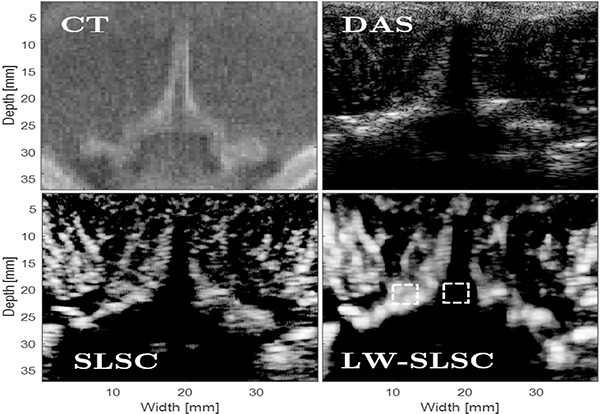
Examples of reconstructed CT, DAS, SLSC (M = 9) and LW-SLSC (*N*_*L*_ = 28, *α* = 0.1) images of the caprine sample (not registered). Regions selected for gCNR measurements are denoted by the dashed boxes.
